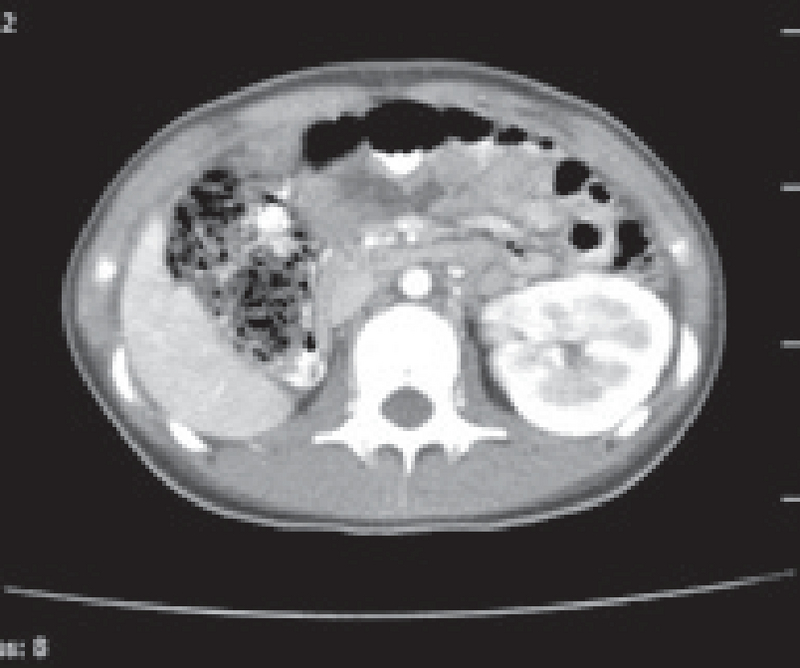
Abdominopelvic CT-scan: multi-cystic mass with severe development in the right adnexa.
